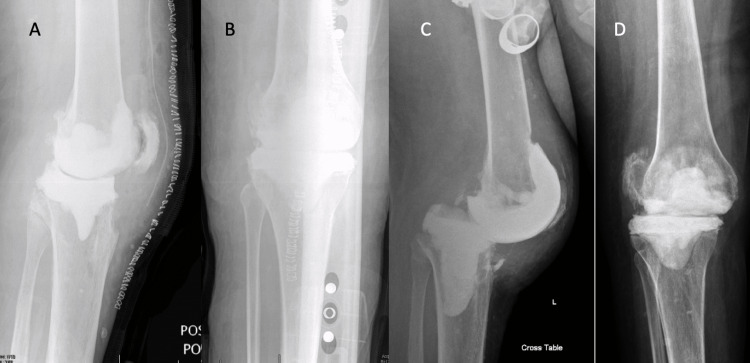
Dynamic knee spacer mechanical complication Immediate postoperative lateral (A) and anteroposterior (B) and six-week postoperative lateral (C) and anteroposterior (D) radiographs demonstrating posterior dislocation of the tibia
